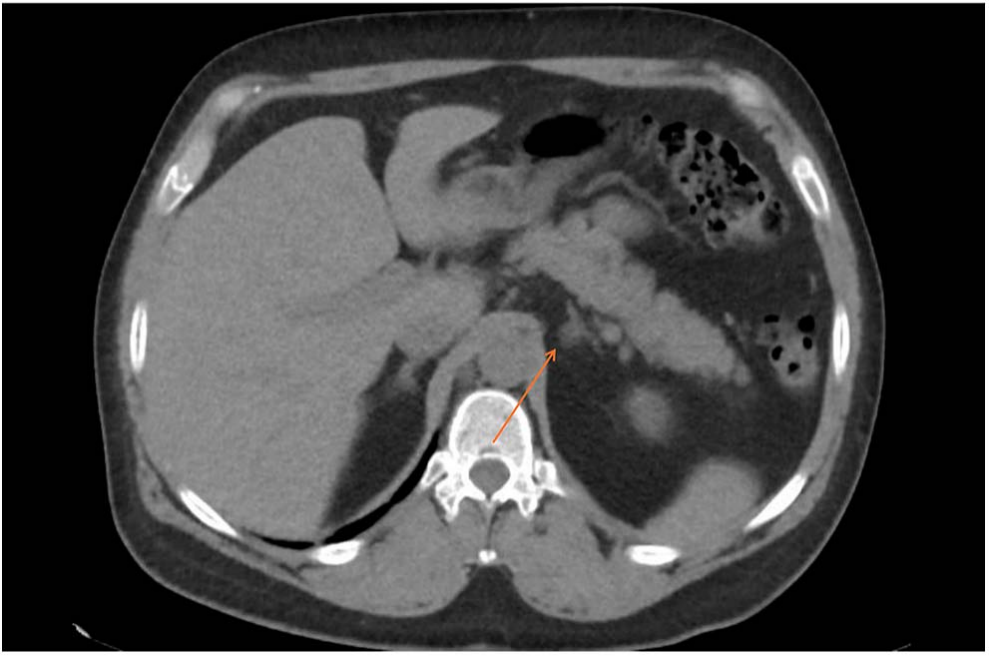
Axial view of CT of the adrenals showing a 1.2 cm left adrenal nodule of 35 houndsfield units.
